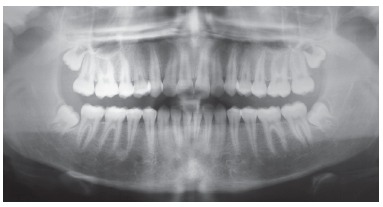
Baseline panoramic radiograph obtained before first orthodontic treatment.
